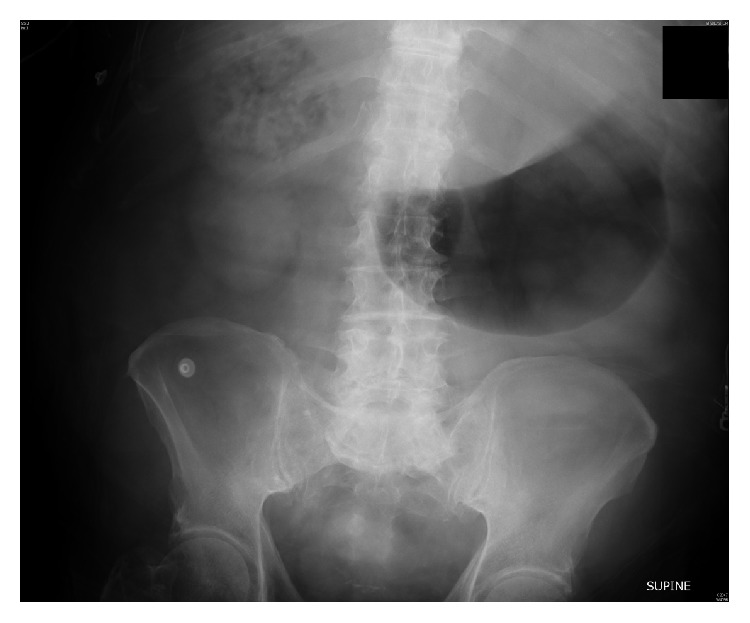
Abdominal radiograph showing right upper quadrant gas bubbles.
